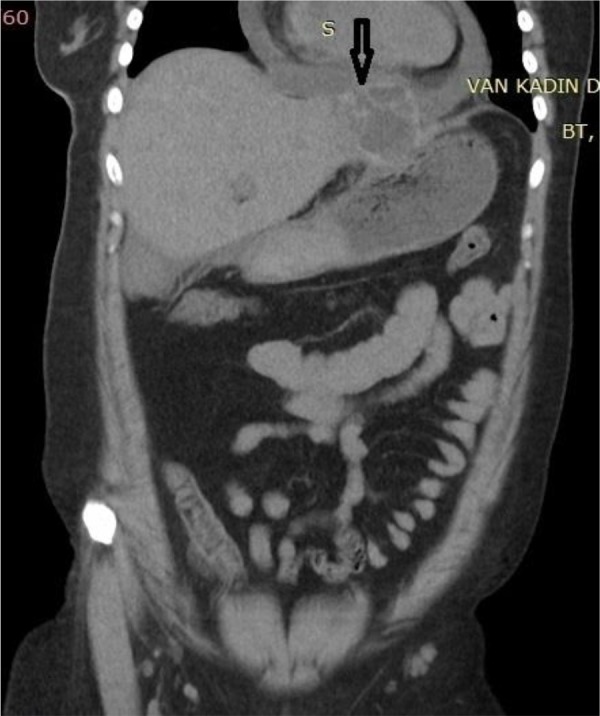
A coronal reformatted CT image at second admission detects a diaphragmatic defect between cyst and pericardial space which is consistent with a trans diaphragmatic fistula (Arrow)
